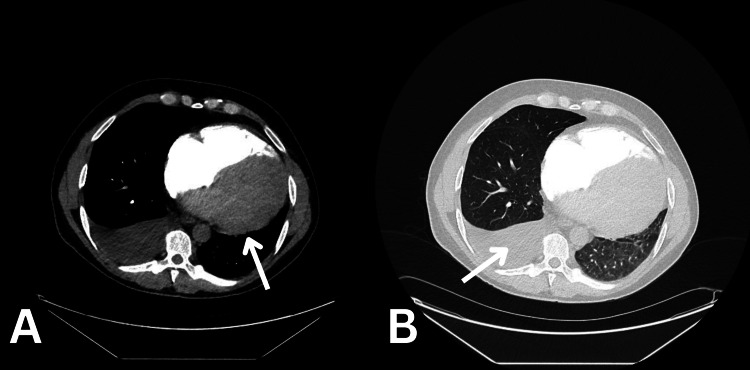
CT-PA soft tissue (A) and pulmonary (B) view. A: The arrow highlights the enlarged and globular heart; B: The arrow points to the right pleural effusion. Note: Contrast reflux is noted to enter into the inferior vena cava and hepatic veins. PA: pulmonary angiogram.
